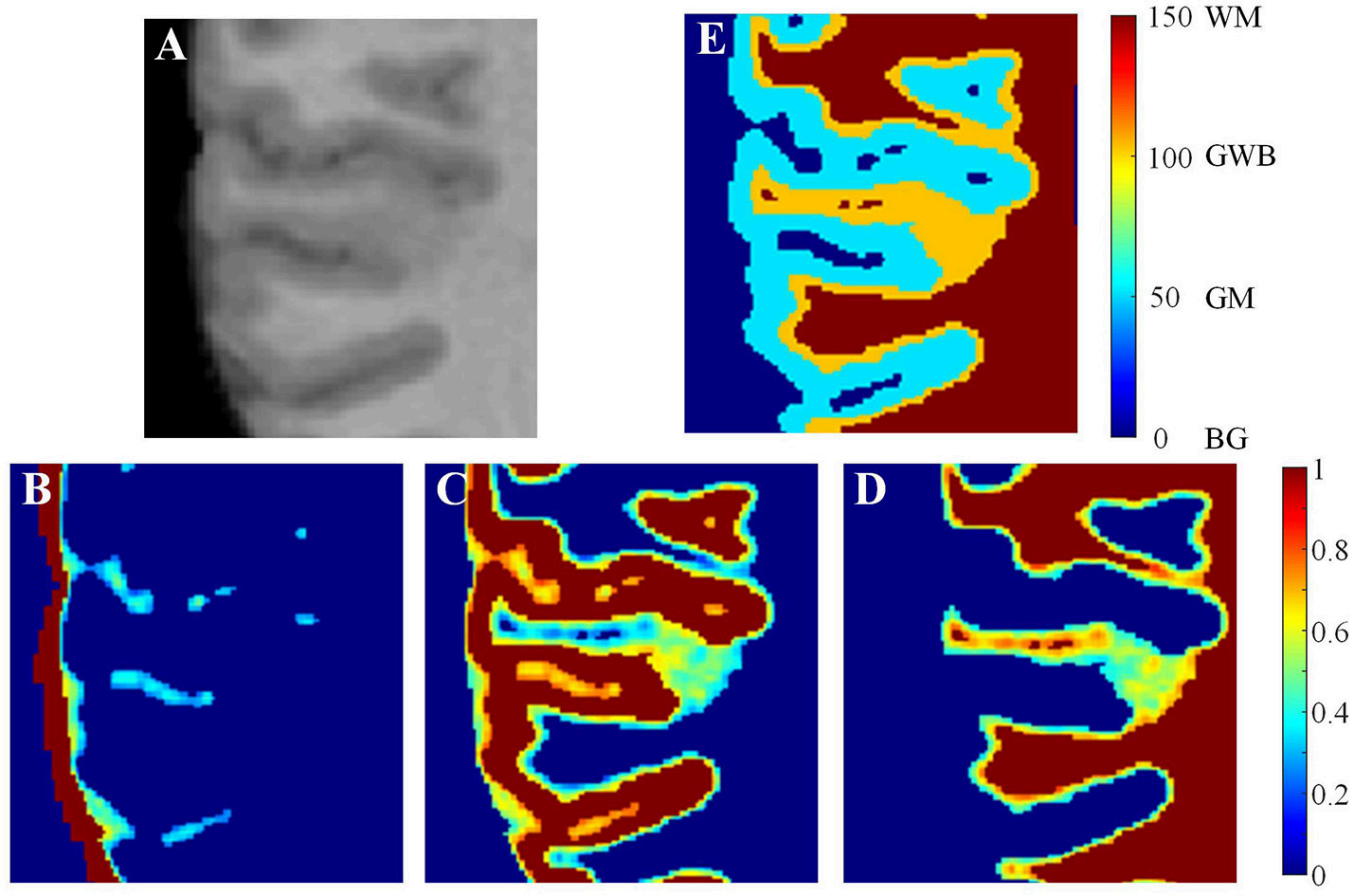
Labeling the regions of interest. In the brain MR image (A), the main tissues are GM, WM, and CSF whose probability images are shown in (B–D). Based on the probability images, the gray/white matter boundary (GWB), white matter (WM), gray matter (GM), and background (BG) are labeled. A small part of an axial slice is enlarged as an example of image taking (A). As a gray scale image cannot present enough detail, we have applied jet color-map to display the images. The image (E) is the labeled image with WM, GM, GWB and background according to the probability images.
